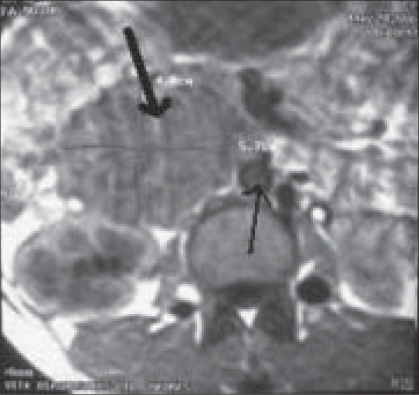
Magnetic resonance imaging of the tumor. Thick arrow – The tumor and the thin arrow – Aorta
